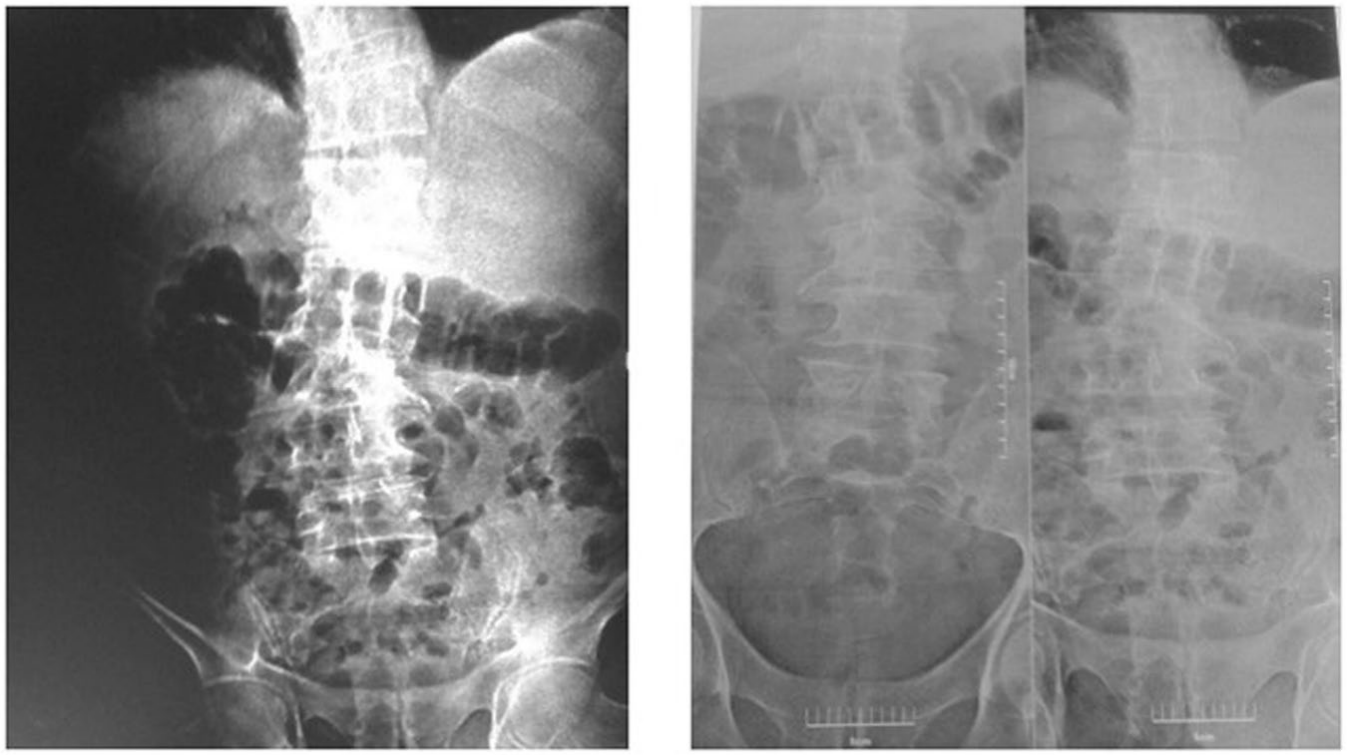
X-ray were referred for bone pain and abnormal findings on radiography.
